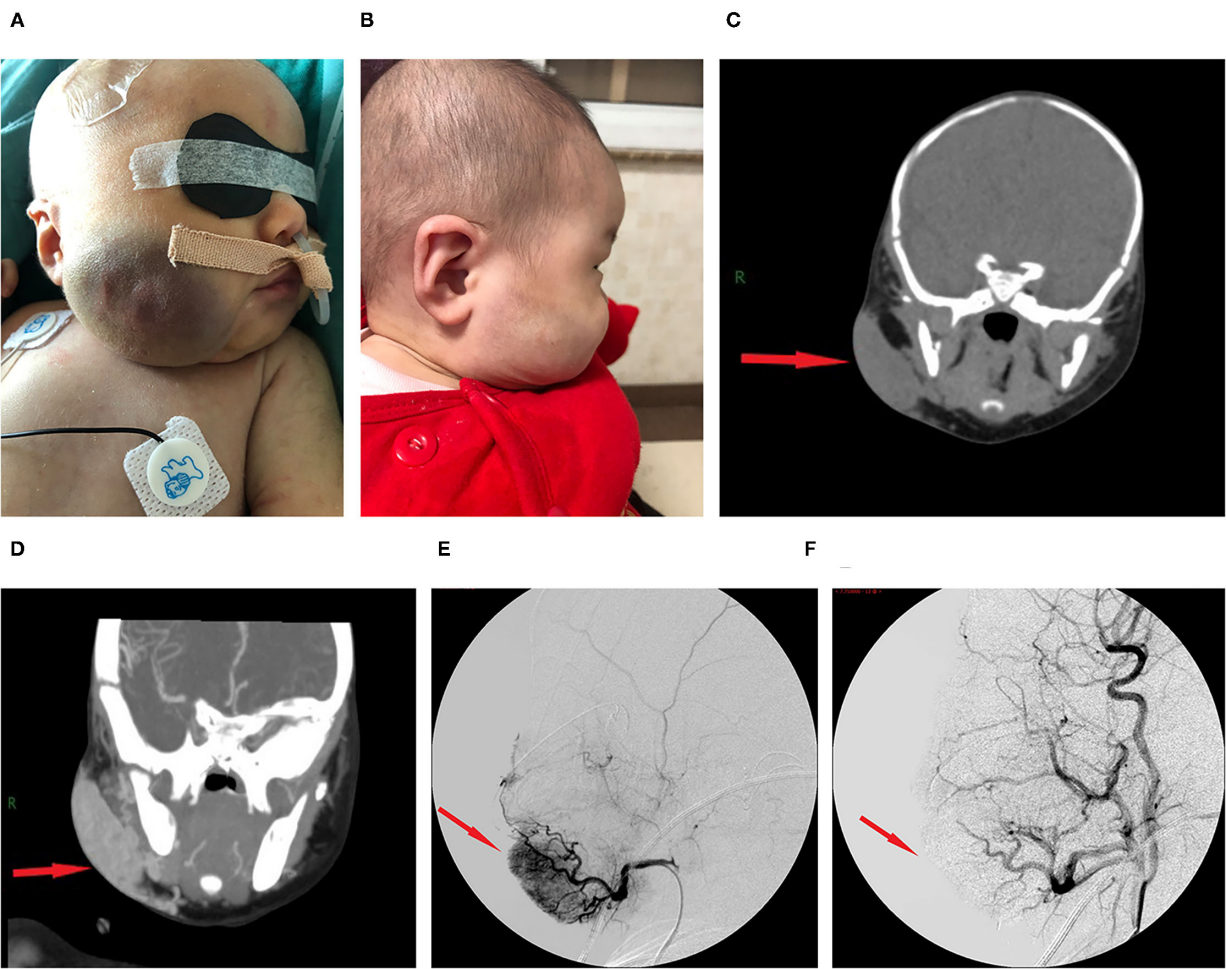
Images from case 8 before and after TAE. (A) A large tumor that measured 7.0 × 6.0 × 3.0 cm was seen on the right face. The skin of the tumor was different from the surrounding area, with purple or dark color, obvious swelling, increased tension, slightly elevated but with ill-defined margins, and induration. (B) At 5 months after TAE, the tumor almost involuted completely. (C) CT showed that the lesion was a large soft tissue mass. (D) Contrast-enhanced CT showed obvious arterial phase enhancement inside the mass. (E) CTA showed an abundant tumor blood supply before embolization. (F) After TAE, more than 80% of the tumor-feeding arteries were embolized. CTA, computed tomography–angiography; TAE, transarterial embolization. The red arrow indicates the lesion.
